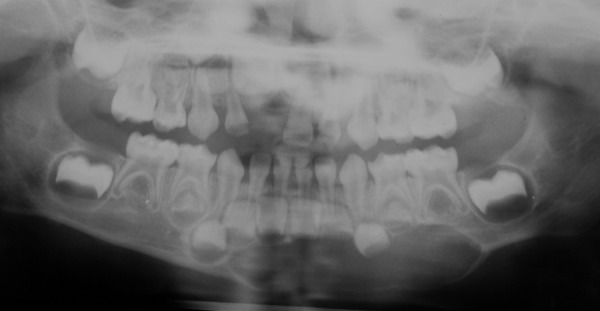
Panoramic radiograph showing a partly well-defined, solitary unilocular radiolucency with scalloped and corticated borders
